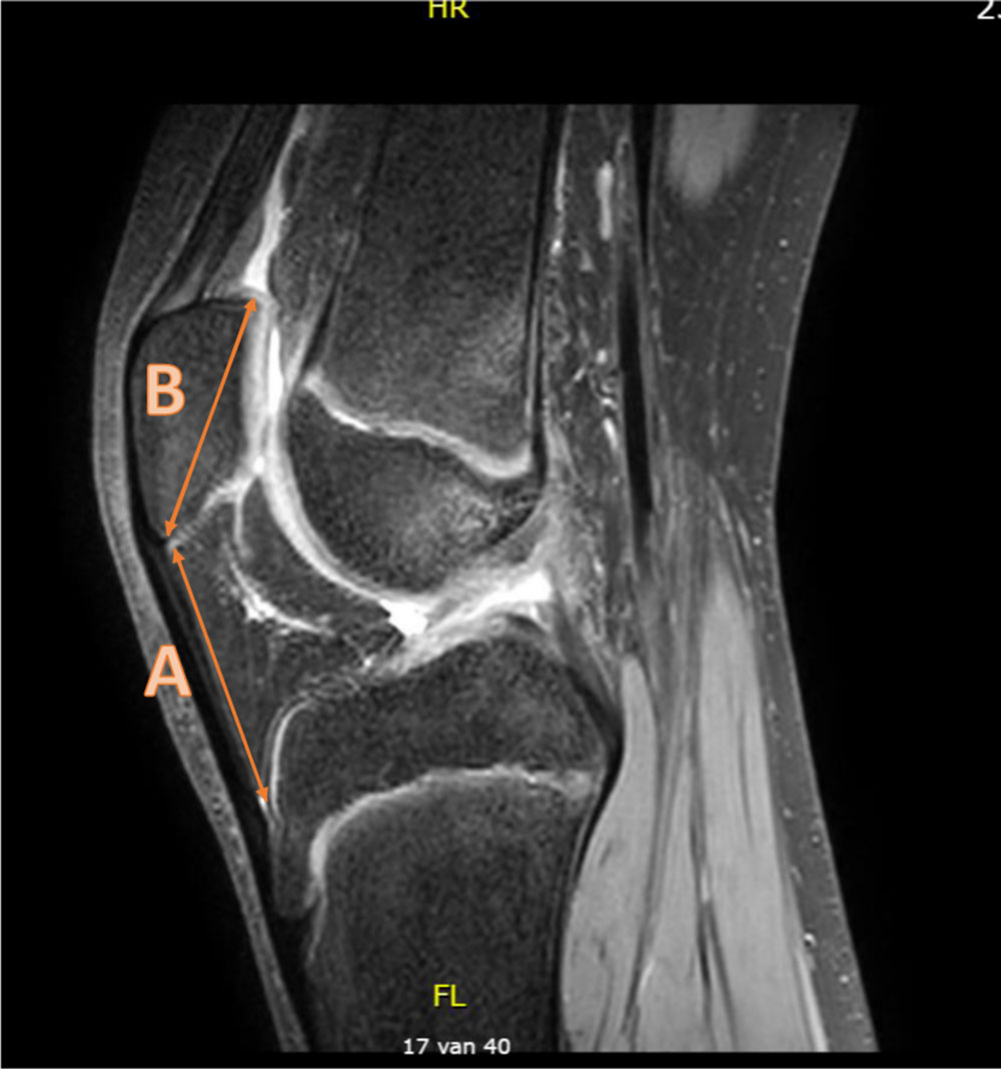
Patellar height measured by Insall–Salvati. This graphical representation shows patellar tendon length measurements (A) and patella length (B) based on MRI examination of knee. Insall–Salvati ratio is calculated from the ratio of A/B [34]. MRI, magnetic resonance imaging.
